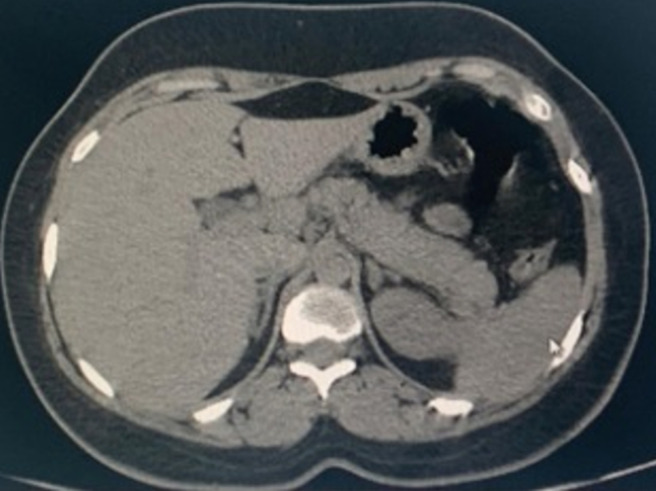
abdominal CT scan in axial section showing a tumefied pancreas with loss of its physiological lobulations associated with an infiltration of peripancreatic fat
